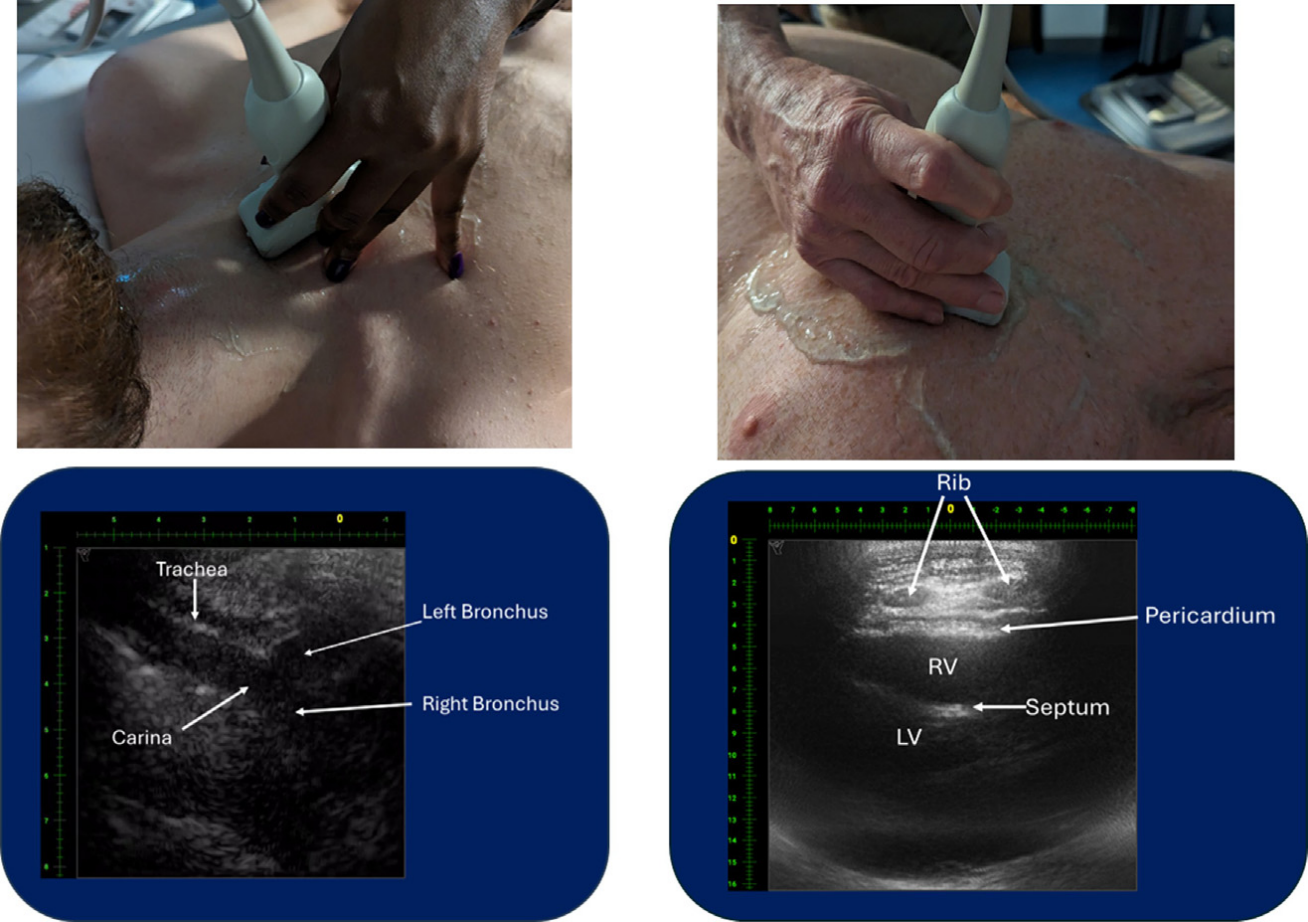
Trachea, bronchi, and heart imaged through the sternum and ribs. The image on the left was obtained by placing the probe in the middle of the upper chest with the probe extending from the suprasternal notch, over the manubrium, and over the upper portion of the body of the sternum. The image on the right was obtained by placing the probe over the left parasternal region and angled along the axis of the heart. This view of the pericardium and heart is significantly easier to obtain with the concave MAUI probe than the subxiphoid view and would be the preferred image for use in the eFAST examination. Note the oval shape of the ribs when viewed in cross-section with no rib shadowing.
